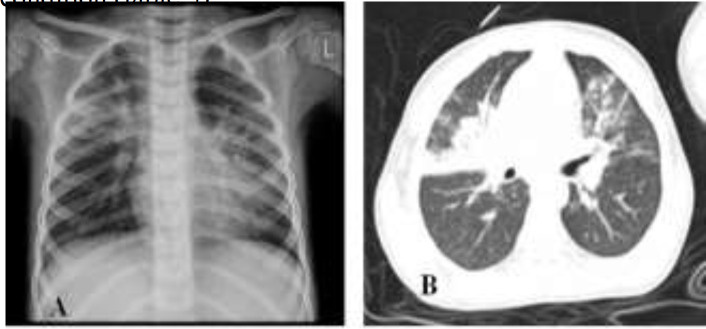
CXR (A) and lung CT (B) showed bilateral airspace consolidation and ground-glass opacity
